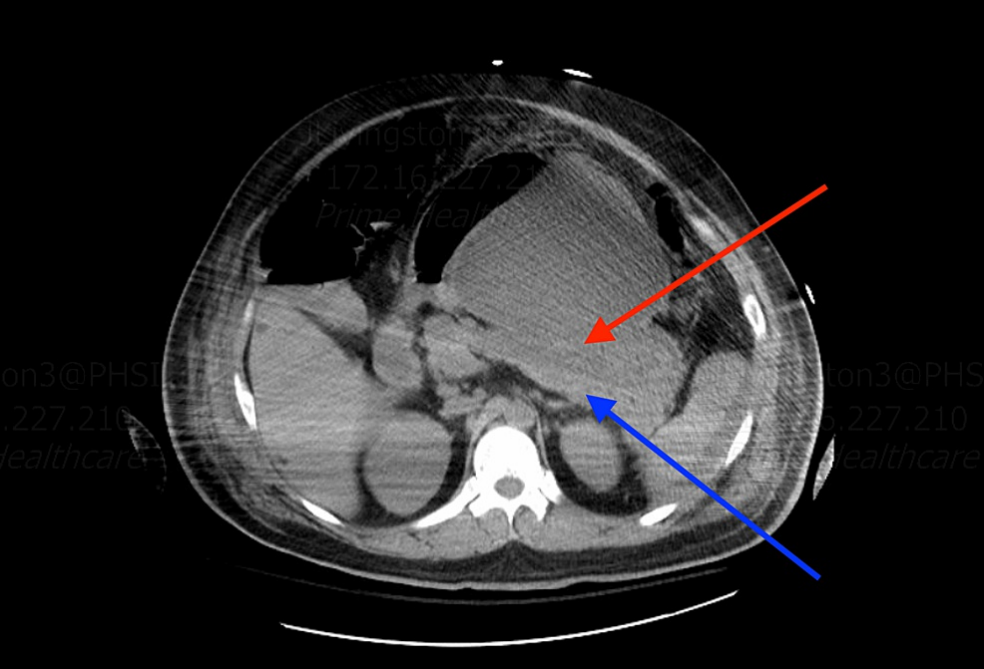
Non-contrast CT scan of the abdomen and pelvis The body of the pancreas appears atrophic (blue arrow). There is a large loculated fluid collection seen around the body and tail of the pancreas abutting the greater curvature of the stomach consistent with pancreatic pseudocysts (red arrow).
